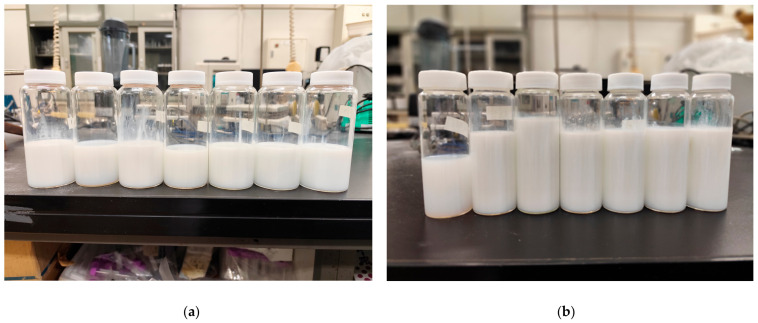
(a). Ultrasonication method on Day 30 and (b). High-pressure method on Day 30.
